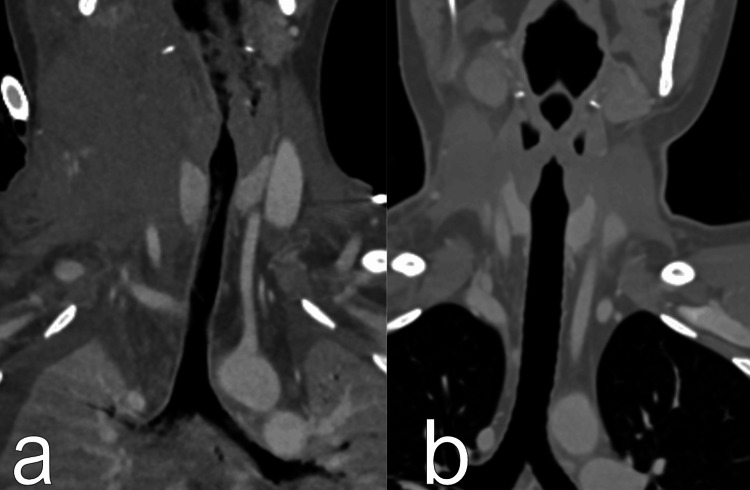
Patient's CT scans a) The CT scan on the day of complication; b) The CT scans one month later
